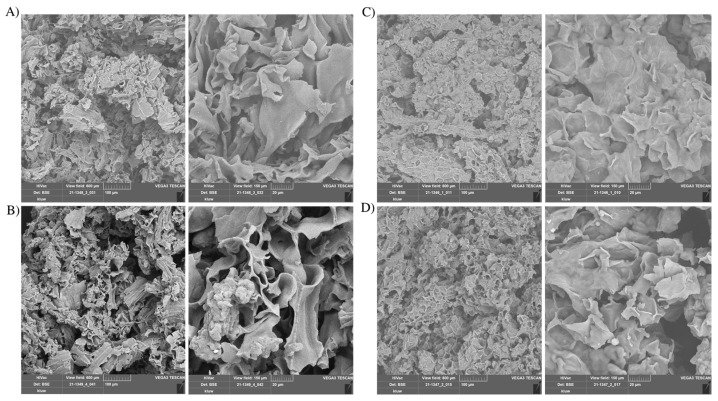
Comparison by the SEM of raw and ultrasound treated press cakes at two different magnifications (left 300× and right 1500×). (A) Raw coconut press cake; (B) ultrasound treated coconut press cake; (C) raw almond press cake; (D) ultrasound treated almond press cake.
